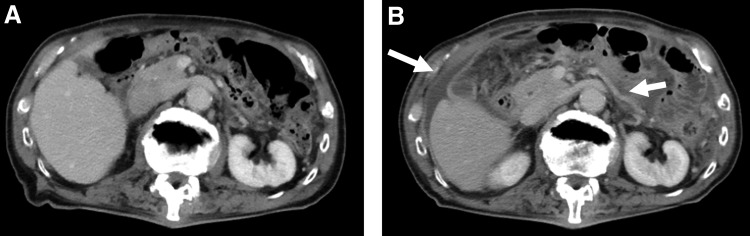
CT findings in the abdomen: (A) preoperative and (B) postoperative. *Arrows* point to the thickened peritoneum.
